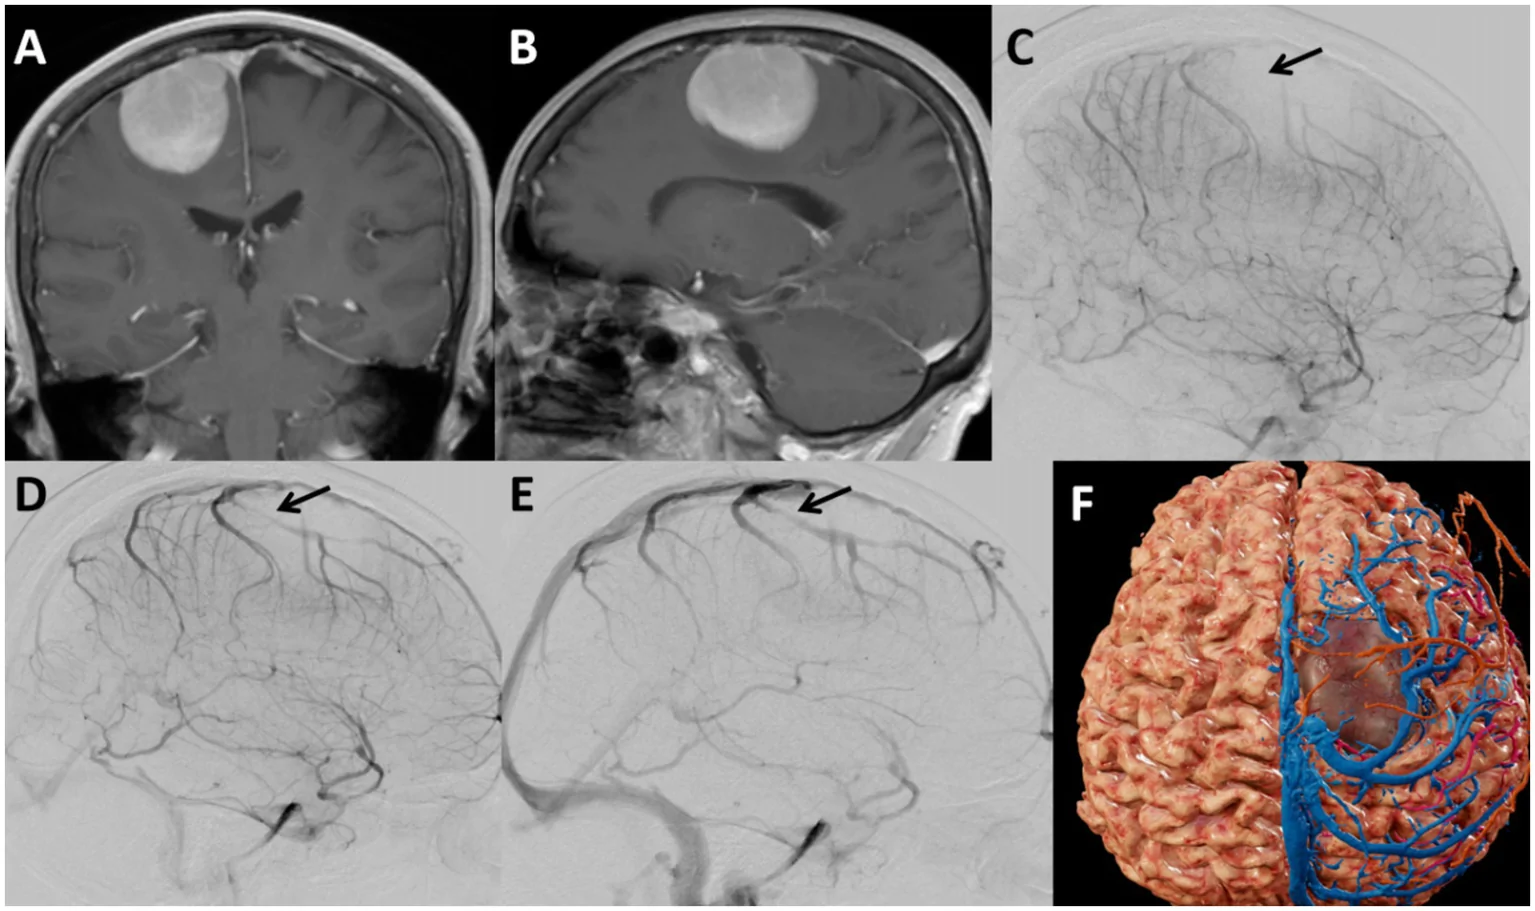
Cortical venous drainage impairment caused by meningioma-related compression. (A,B) Preoperative MRI showing the lesion and its relationship with adjacent cortex. (C–E) Venous-phase DSA demonstrating impaired cortical venous drainage surrounding the tumor. The compressed cortical veins are indicated by black arrows. (F) MFI delineating the course of the cortical veins, which were identified and preserved during surgery.
